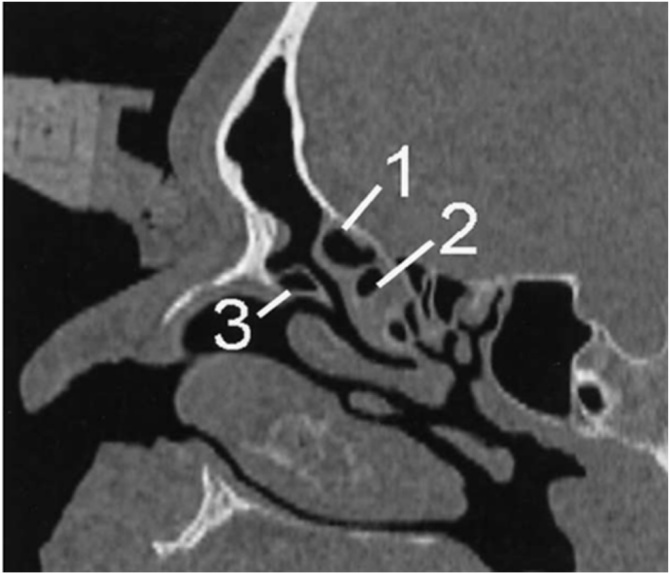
Bulla ethmoidalis. Sagittal computed tomography section demonstrates supra bulla cell (1) that is above the bulla ethmoidalis (2). An Agger nasi cell (3) is also shown.
